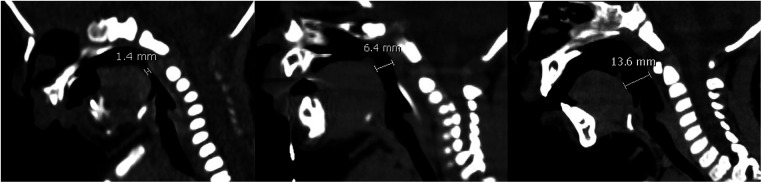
From left to right are CT images on preoperative day 1, postoperative day 28, postoperative 1 year 2 months. CT images showed the distance between the base of tongue and posterior pharynx wall was 1.4 mm, 6.4 mm, 13.6 mm.
